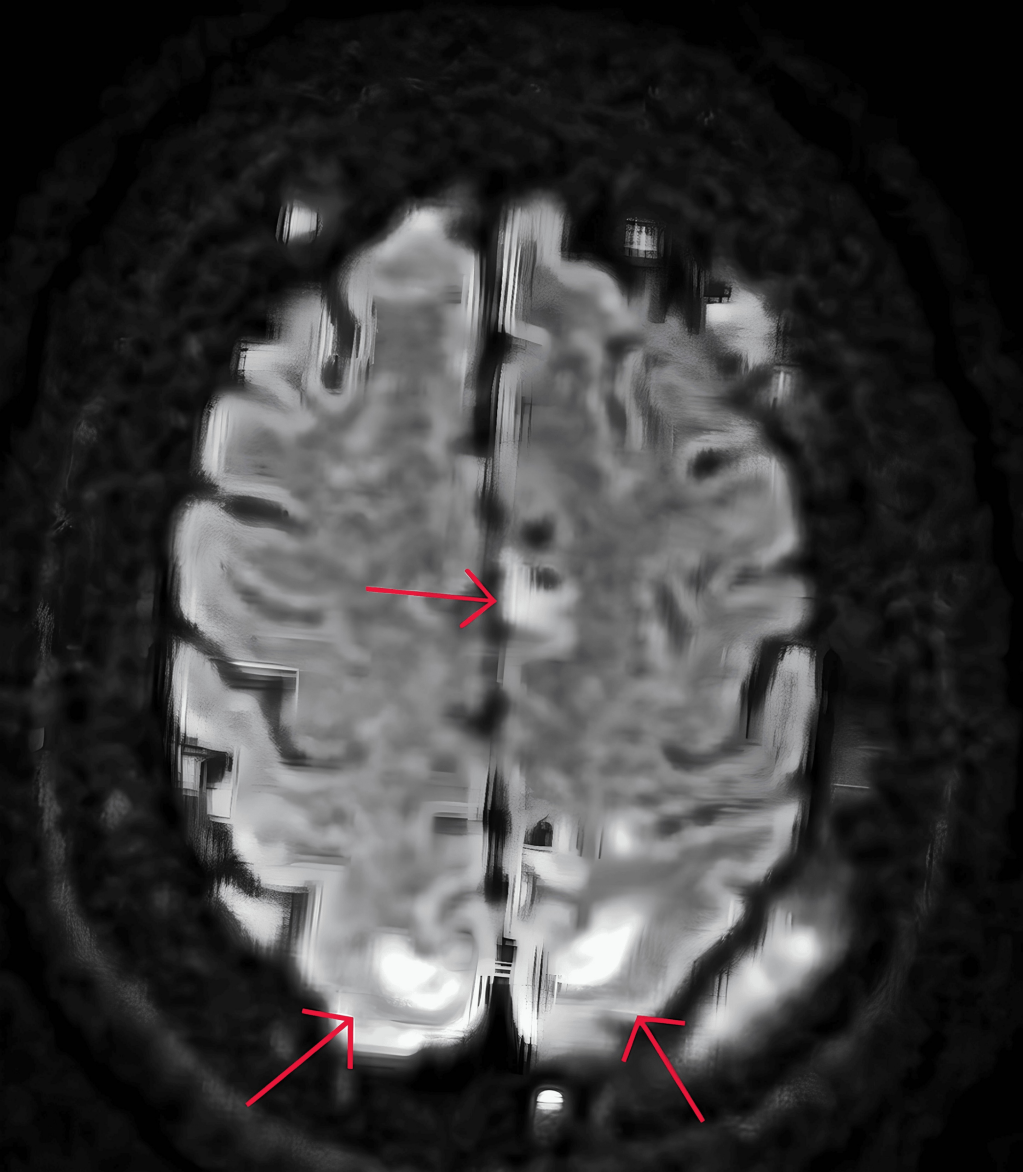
MRI axial DWI slice Multifocal areas of high-signal intensity are seen in the bilateral parieto-occipital lobes DWI: diffusion-weighted imaging
